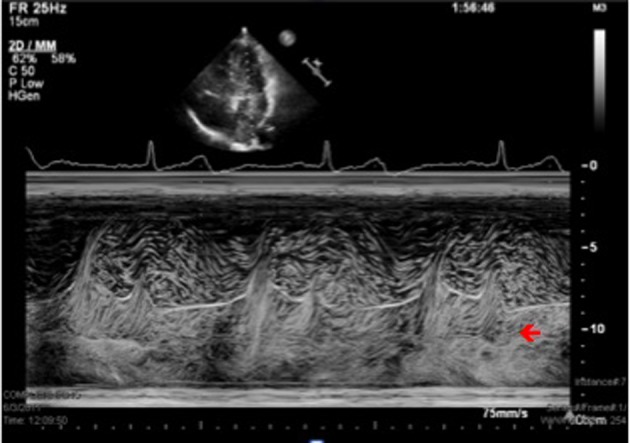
M Mode Echocardiography through the left atrium and left ventricle showing bubbles after agitated saline injection in the left arm.
